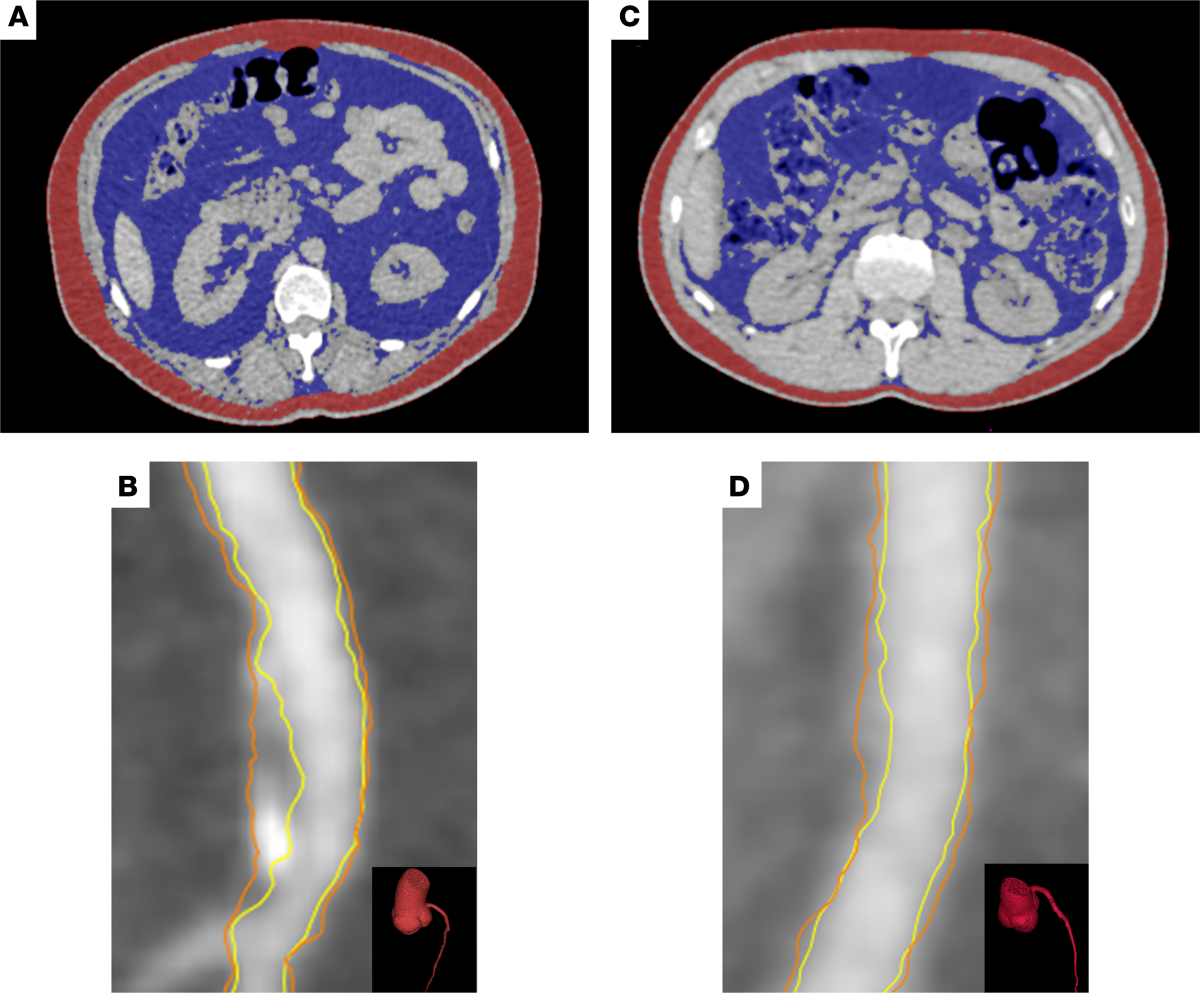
Visceral adiposity and noncalcified coronary artery burden in psoriasis. (A) Axial abdominal CT of a patient with psoriasis with high VAT volume (VAT, 32,767 cc^3^) for quantification of visceral (blue) and subcutaneous (red) adiposity. (B) CCTA showing the proximal left anterior descending artery (LAD) of the same patient, with the lumen (yellow) and the outer walls (orange) (LAD noncalcified coronary artery burden, 1.04 mm^2^) shown. (C) Axial abdominal CT of an age- and sex-matched patient with psoriasis with low VAT (VAT, 13,900 cc^3^) for quantification of visceral (blue) and subcutaneous (red) adiposity. (D) CCTA showing the LAD of the same patient, with the lumen (yellow) and the outer wall (orange) (LAD noncalcified coronary artery burden: 0.84 mm^2^) shown.
